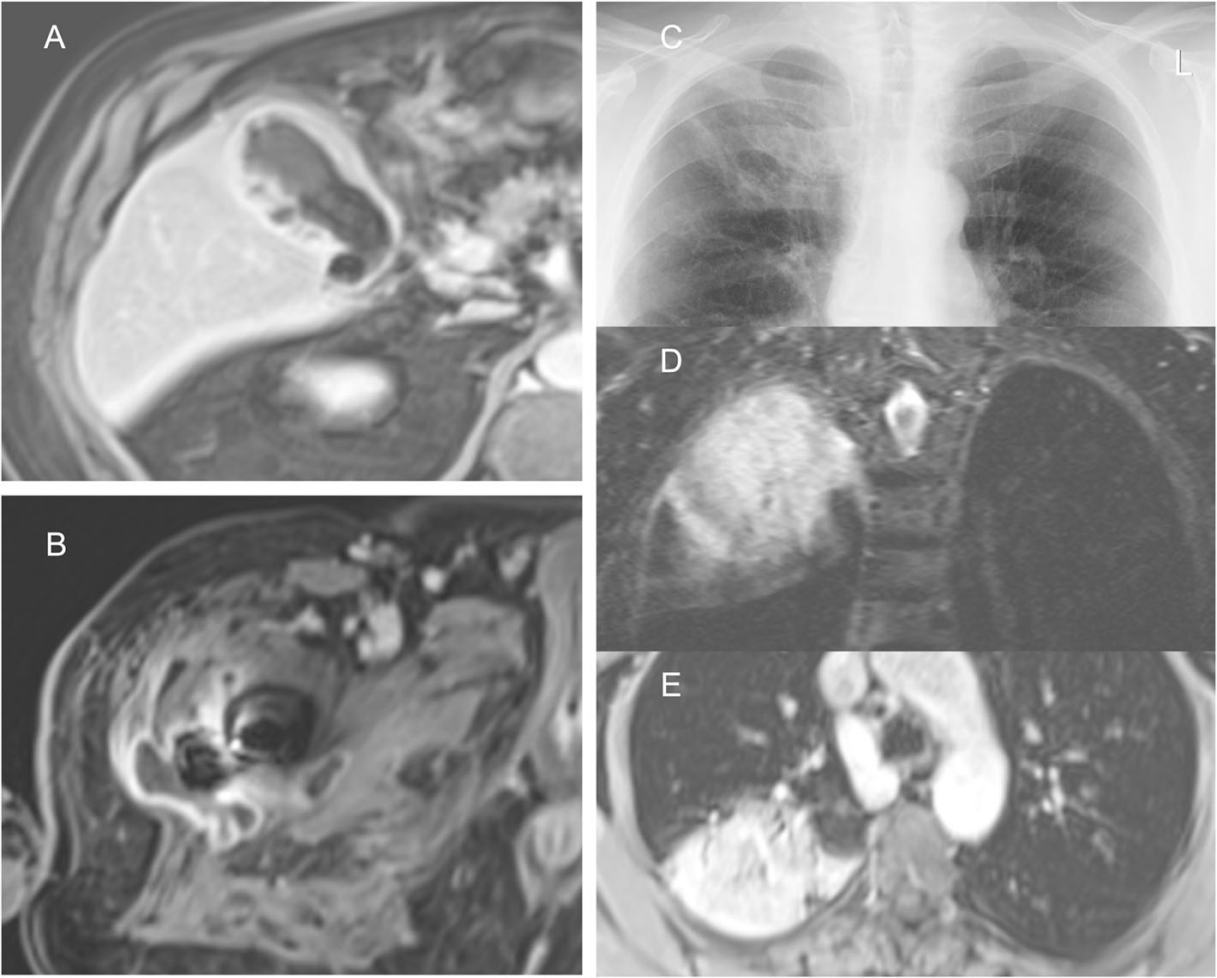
Infectious foci found by wb-MRI in patients with FUO. Axial contrast enhanced T1-VIBE image of a 81-year old-patient a shows gallbladder wall thickening, contrast enhancement and lithiasis as signs of subacute cholecystitis which had eluded diagnosis by abdominal ultrasound. In another patient, an 88-year-old female, b the contrast enhanced T1-VIBE displays a perifemoral intramuscular abscess formation as infectious focus which remained occult after standard clinical work-up. Conventional chest radiography c, coronal T2-STIR sequence d and axial contrast enhanced T1-VIBE e of a 67-year-old patient with right upper-lobe pneumonia. The radiography shows discrete reticular opacities in the right upper zone suggesting pulmonary infiltration while T2-STIR and contrast enhanced T1-VIBE prove right upper lobe pneumonia as infectious focus. The radiography was taken 1 day prior to the wb-MRI
